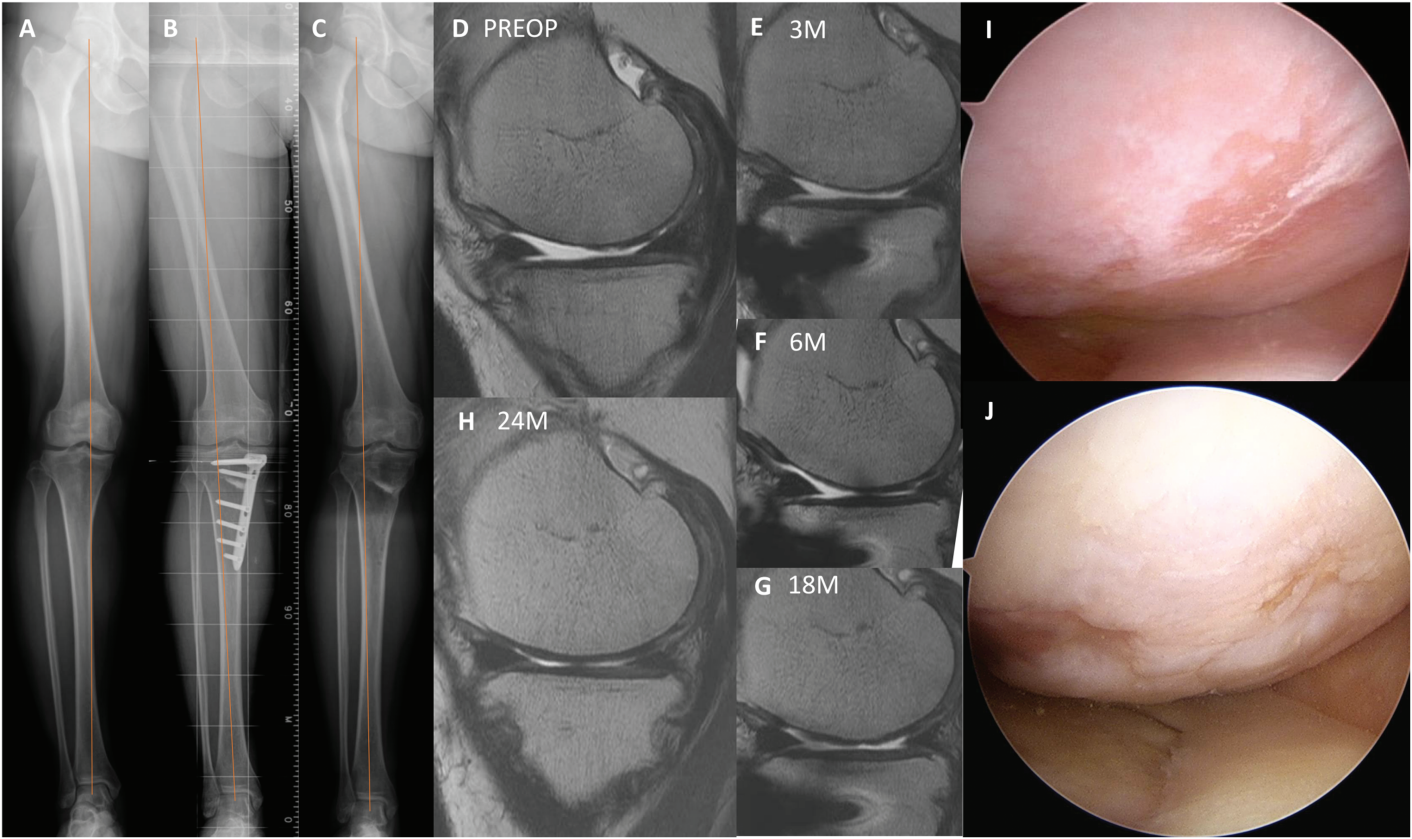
Representative case of MRI and arthroscopic changes after MOWHTO with intra-articular injection of ADMSCs. Preoperative medial compartment OA with varus alignment (A) of the right knee of a 50-year-old female patient is corrected to valgus alignment via MOWHTO (B) and maintained well at postoperative 2 years (C). Preoperative T2-weight sagittal image of MRI shows a full-thickness defect of articular cartilage in the MFC (D). Cartilage regeneration along favorable integration with adjacent native cartilage is observed through serial MRI follow-up up to postoperative 24 months (E-H). Arthroscopic findings of cartilage status in MFC show the exposure of subchondral bone at the time of initial arthroscopy (I) and nearly total coverage of regenerated cartilage 2 years after intra-articular injection of ADMSCs to MOWHTO (J). Abbreviations: ADMSC, adipose-derived mesenchymal stem cell; MFC, medial femoral condyle; MOWHTO, medial open-wedge high tibial osteotomy; MRI, magnetic resonance imaging; OA, osteoarthritis.
